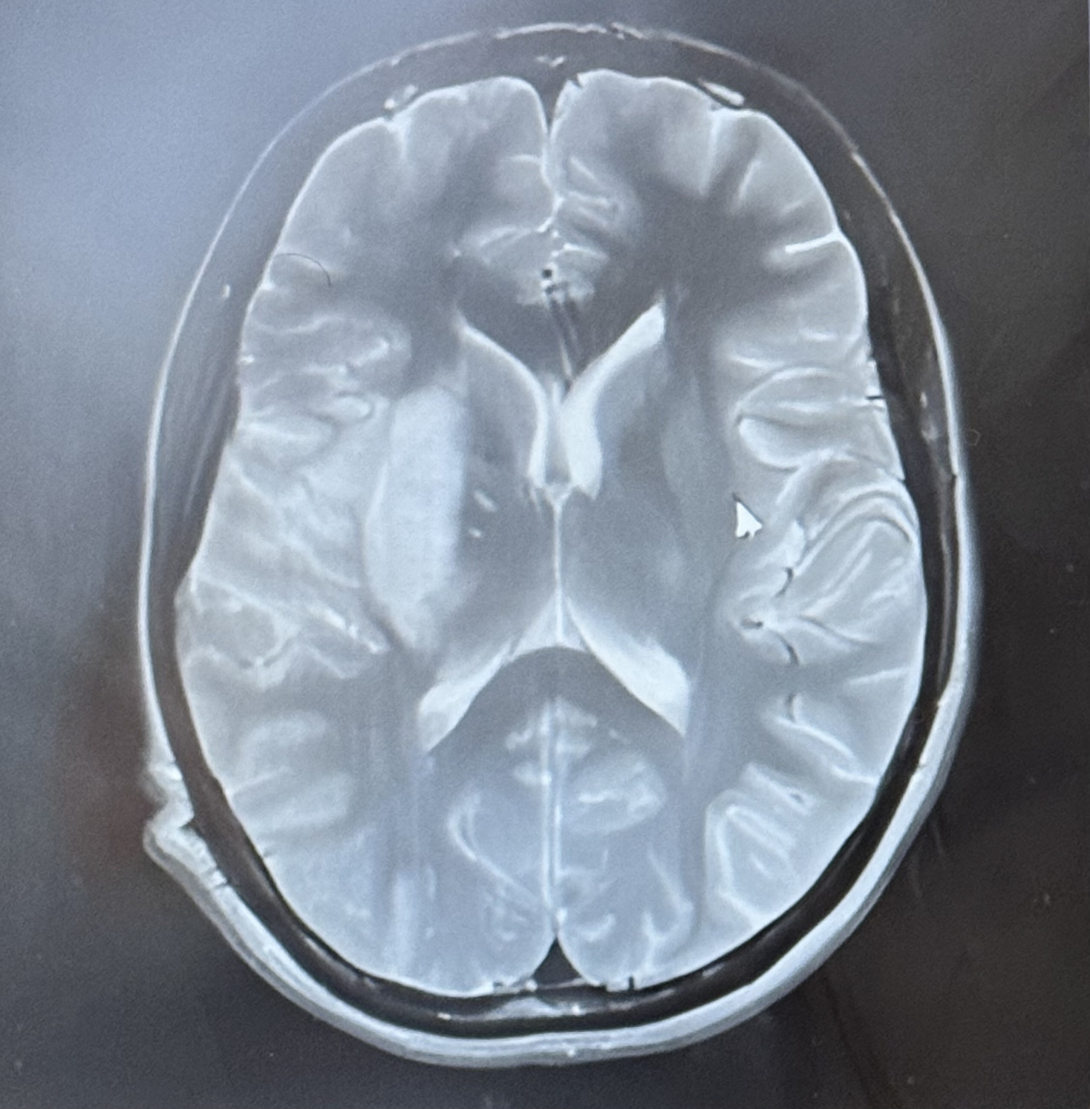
Brain MRI showing an ischemic stroke in the deep territory of the right middle cerebral artery
